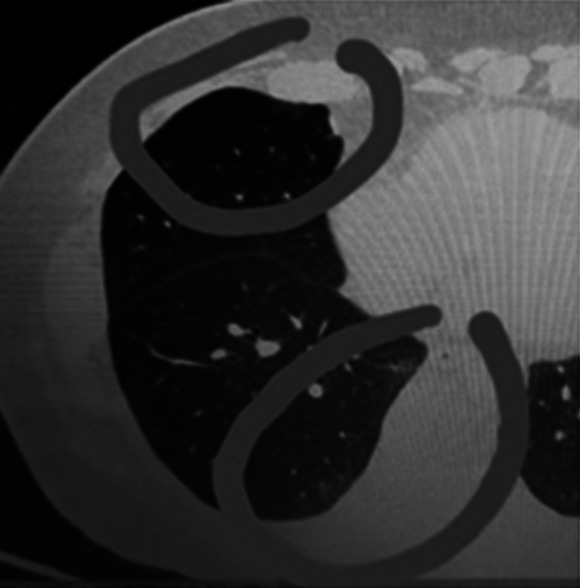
CT-scan chest/lung with 128 slices of the patient on the 12th day of treatment. Circles indicate ground-glass appearance on the right lobe lung.
